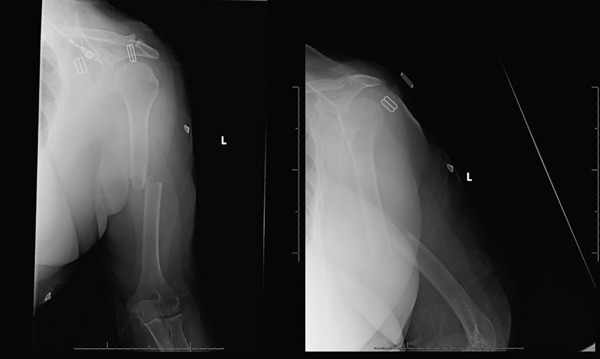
AP and lateral radiographs of the left humerus at time of initial presentation to the emergency department. Radiographs demonstrate a left midshaft humerus fracture with bayonet apposition and approximately 35° of apex anterior angulation.
